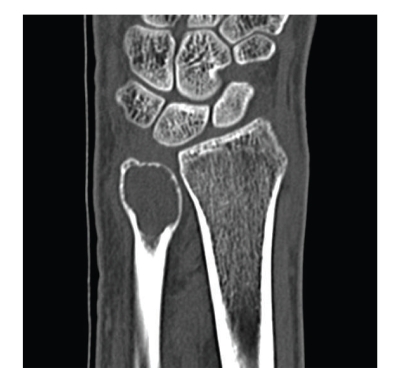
Computed tomogram showed thinning and protrusion of the cortex but no destruction of the cortex of the distal ulna.
